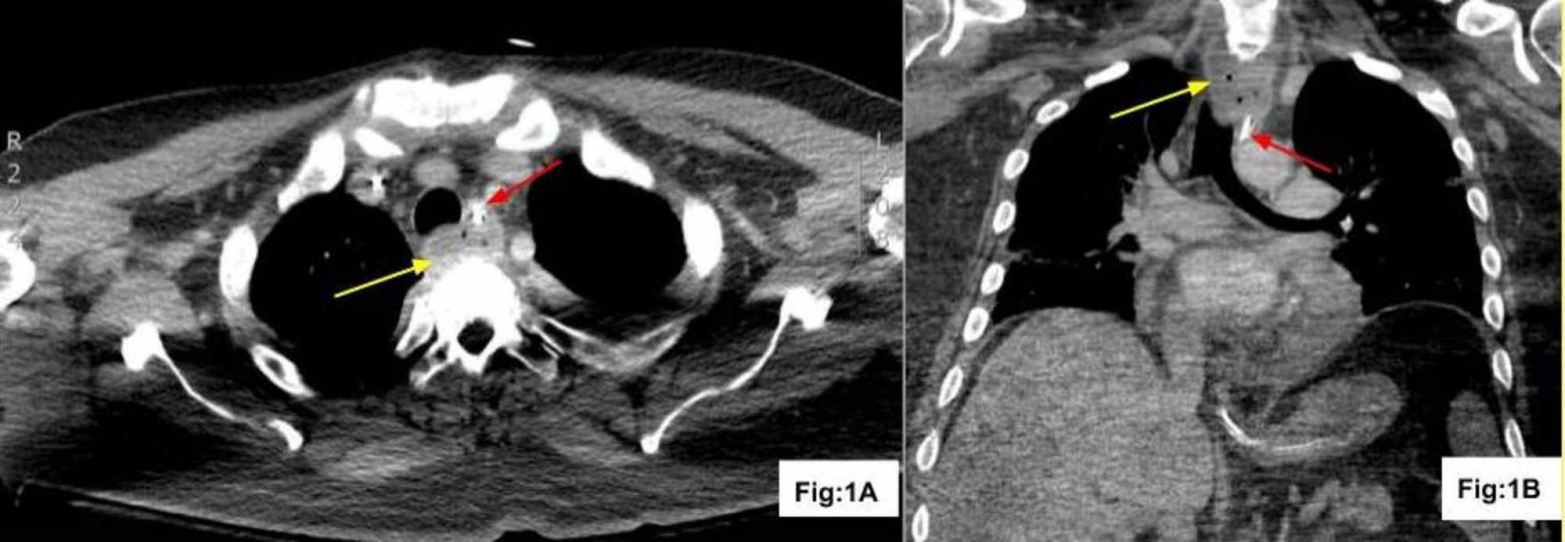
CT of the chest after vancomycin IV treatment CT with contrast axial (Fig: 1A) and coronal (Fig: 1B) reconstruction demonstrates intramural esophageal abscess with tiny gas locules (yellow arrow) displacing the enteric tube anteriorly (red arrow) CT: computed tomography; IV: intravenous
